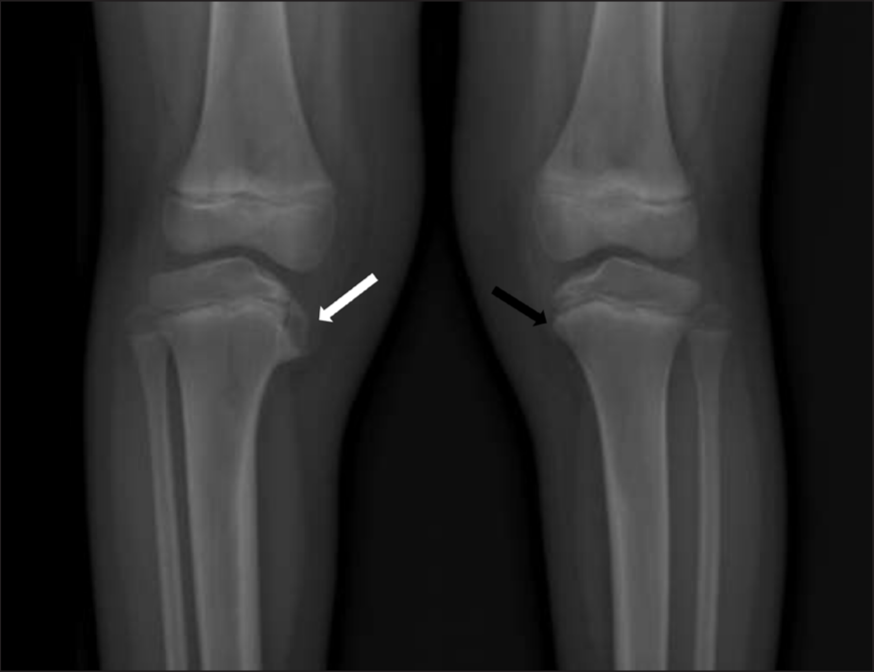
Proton density-weighted magnetic resonance imaging (MRI) on coronal plane (*Figure 2*) showed an abnormal osteochondral maturation of the proximal medial tibial plateau and the adjacent metaphysis. Epiphyseal and metaphyseal cartilage last unossified and distorted. Islands of anarchic bone ossification (*Figure 2*, white arrow) corresponds to the “beak-like appearance” visible on the radiograph. The growth plate appeared normal on MRI except for its medial third on the medial tibial plateau, which was slightly concave and thinned. Medial femoro-tibial joint space was enlarged (*Figure 2*, black arrowhead). The interposed medial meniscus seemed thickened and globally of high signal with no visible lesion.
